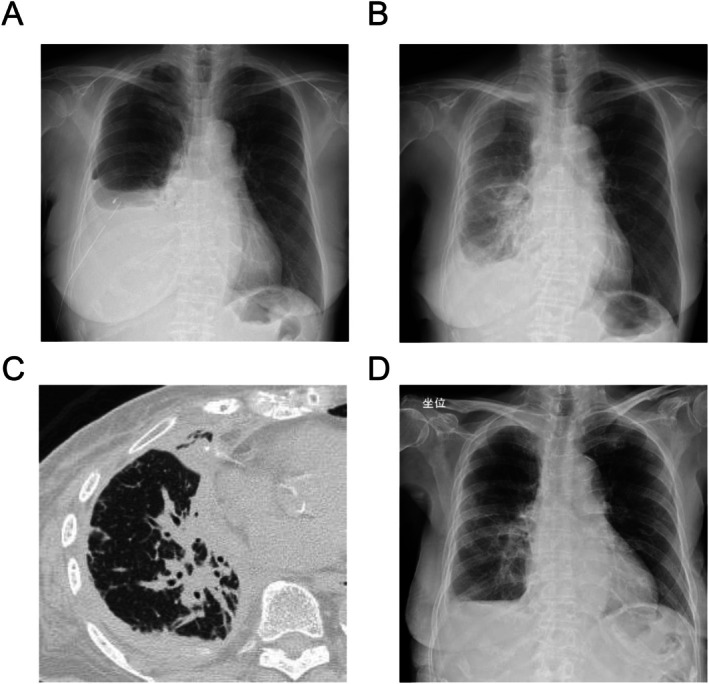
Serial imaging findings (chest radiography and CT). (A) Chest radiography at the time of postoperative recurrence showing a large right pleural effusion consistent with pleural dissemination. (B) Chest radiography obtained immediately before initiation of dabrafenib and trametinib, showing re‐accumulation of right pleural effusion and increased opacity in the right lower lung. (C) Chest CT demonstrating right pleural effusion with perihilar‐predominant infiltrative opacities and bronchial wall thickening in the right lung, findings suggestive of pulmonary lymphangitic carcinomatosis. (D) Chest radiography obtained after initiation of reduced‐dose dabrafenib and trametinib, showing a decrease in right pleural effusion and improvement in lung opacity.
